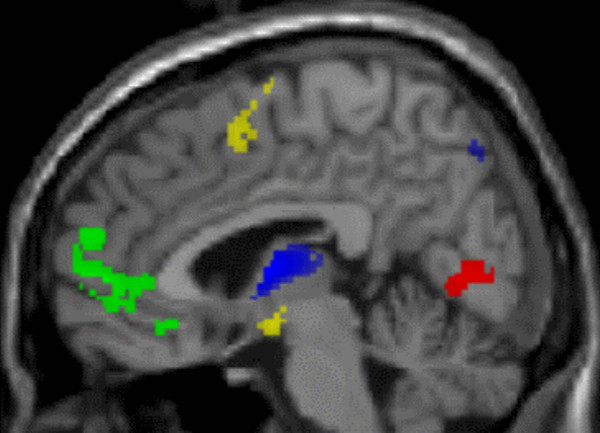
Brain areas involved in the PC1 and PC2 superimposed on the canonical single-subject MR image of SPM2 in a sagittal plane showing the areas involved in PC1 (cuneus: red – negative loading; anterior portion of superior frontal gyrus: green – positive loading) and in PC2 (precuneus, thalamus: blue – negative loading; pre-SMA, hypothalamus: yellow – positive expression loading).
